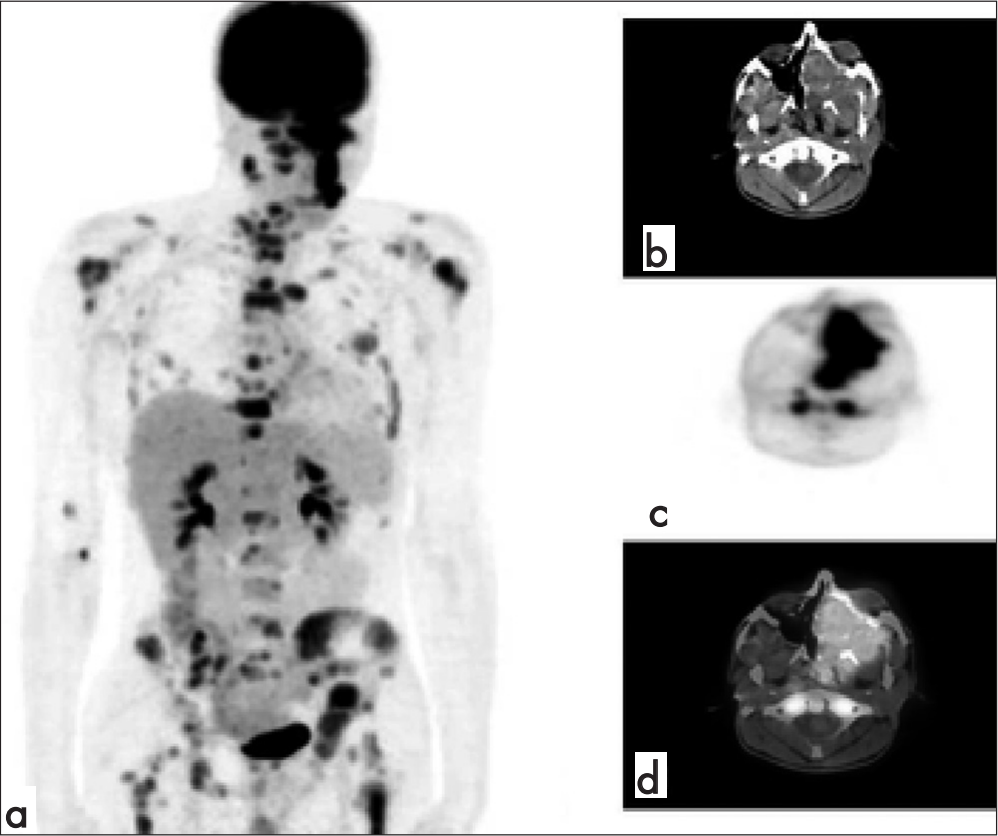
MIP image (a) and transaxial images (b,c,d) of PET/CT revealed the primary lesion (SUVmax: 9.82) and bilateral cervical lymph nodes (SUVmax 9.44), pelvic bones, bilateral proximal femora, bilateral humeri, scapula, sternum, clavicles, ribs, and vertebrae
